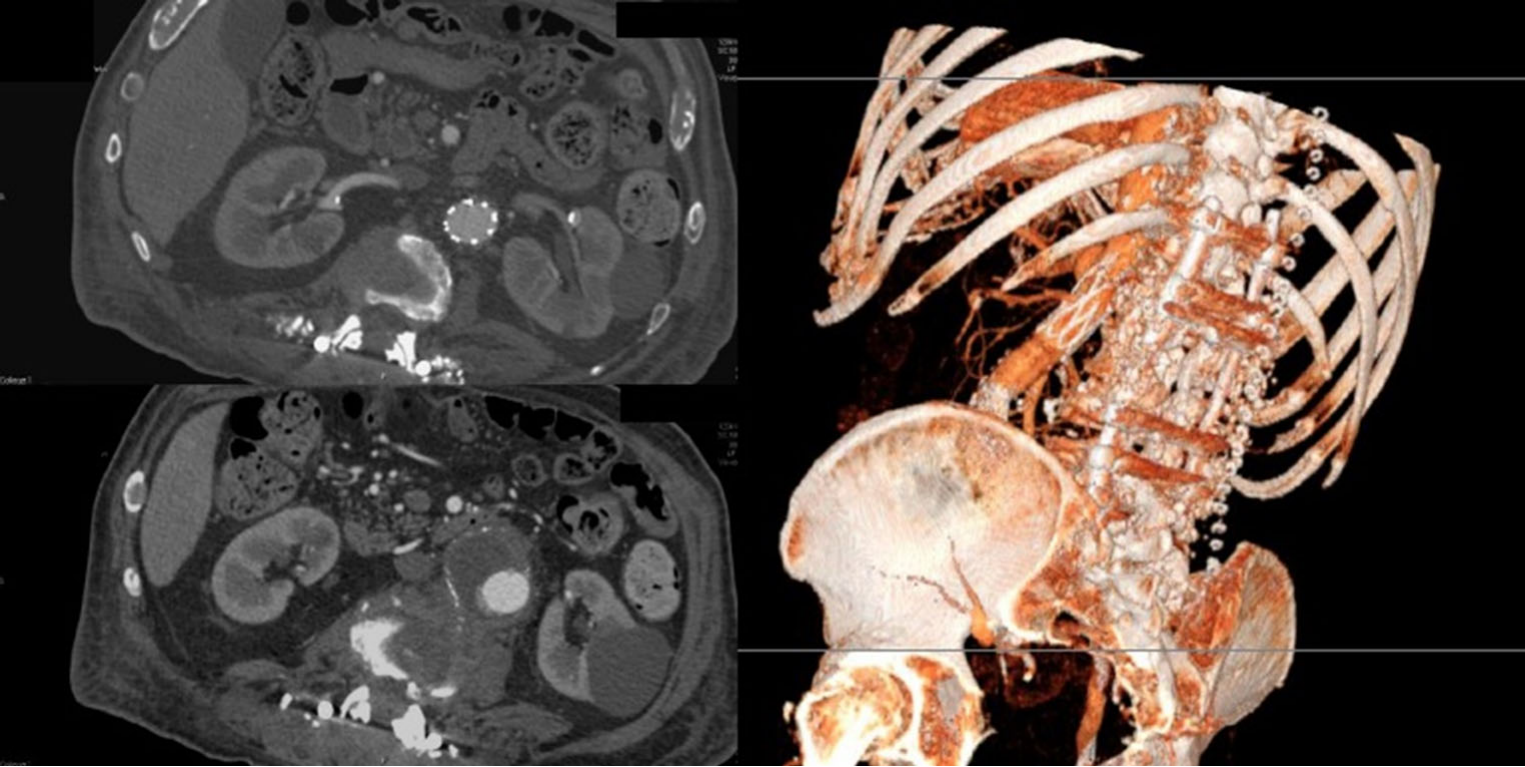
CT scan performed after spinal surgery showing the absence of infra/suprarenal endoleak
